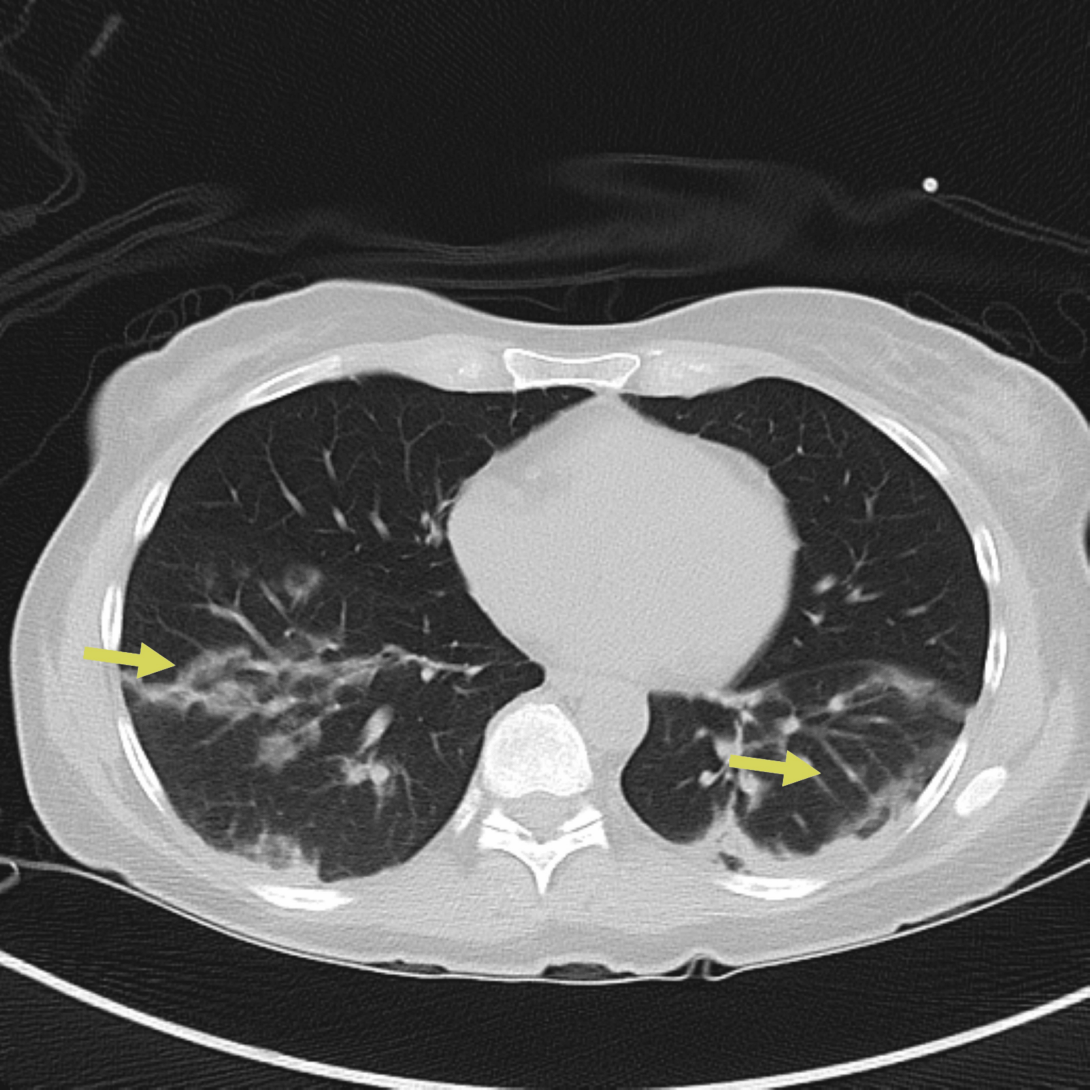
Repeat CT chest on day three of hospitalization showing worsening mixed consolidative and ground-glass opacities, particularly on the left lung (yellow arrows)
